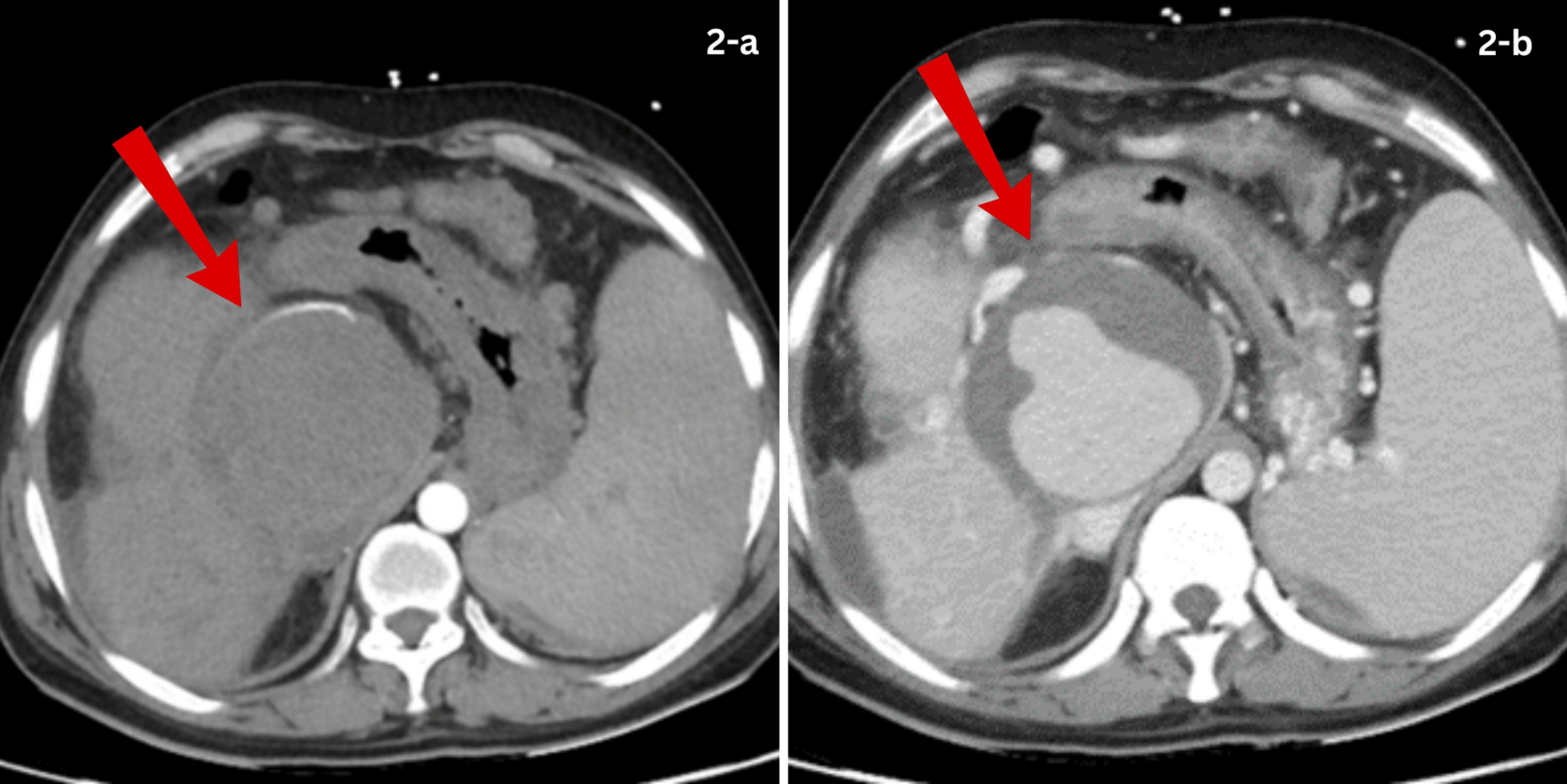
CT images of the hepatic artery pseudoaneurysm. (a) A large right hepatic artery pseudoaneurysm (7.3 x 6.8 cm) displacing the hepatic artery and demonstrating flow in the pseudoaneurysm. (b) Portal venous phase image of the hepatic artery pseudoaneurysm with partial thrombosis of the pseudoaneurysm.
